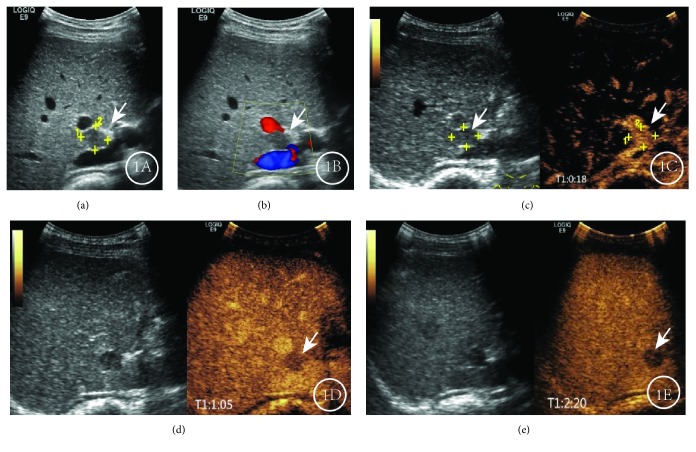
Results for a male patient, aged 46, in whom HCC recurred at 6 months after curative surgery. (a) Routine ultrasound showed hypoechoic nodules (1.8 × 1.5 cm) in the right anterior lobe near the secondary porta of liver. (b) Color Doppler flow imaging (CDFI) showed no color flow signal around or inside the nodule. (c) CEUS showed rapid, uniform enhancement in the arterial phase. (d) CEUS showed a low, rapidly decreasing enhancement in the portal phase. (e) CEUS showed low enhancement in the delayed phase. This CEUS expression pattern was “fast-in, fast-out”.
